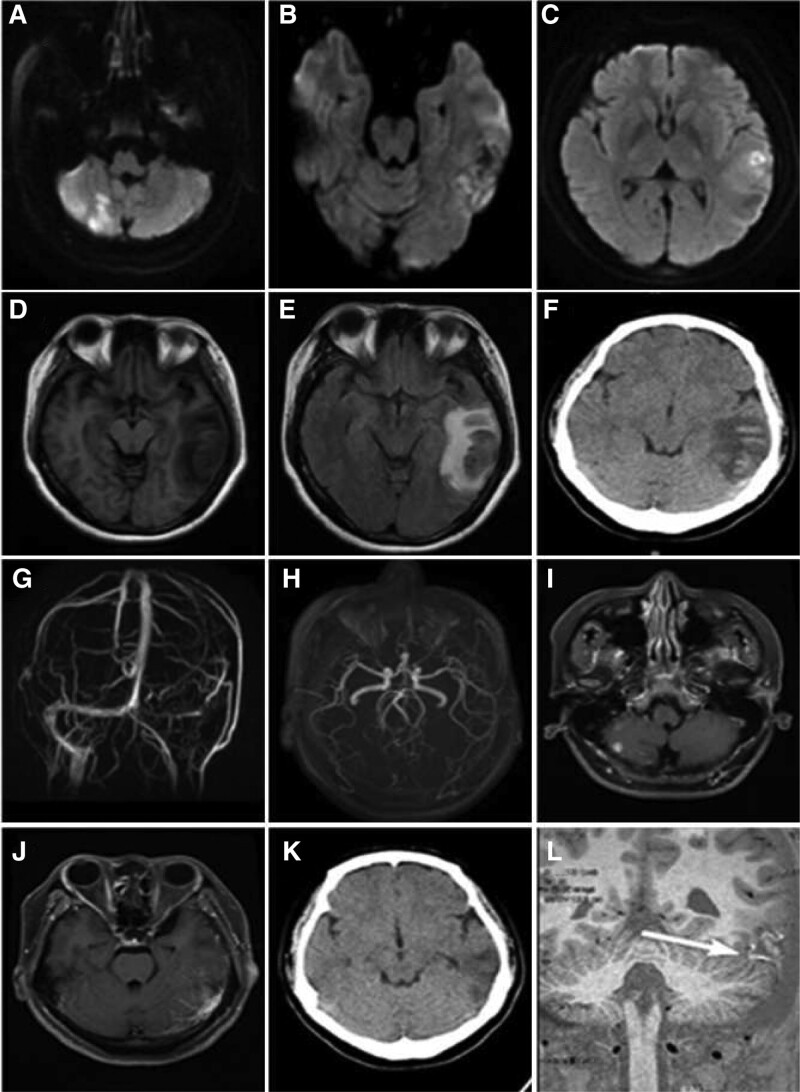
Brain imaging findings before and after treatment. (A, B and C) Diffusion-weighted imaging showed multiple infarcts. (D and E) T1-weighted images (T1WI) and Fluid-attenuated inversion-recovery (FLAIR) images showed heterogeneous signal and distinct edema in the left temporal lobe. (F) CT scan showed hemorrhagic infarction in the left temporal lobe. (G) Magnetic resonance venography (MAV) demonstrated a vague left sigmoid sinus. (H) Magnetic resonance angiography (MRA) showed no arterial vascular stenosis or occlusion. (I and J) Cranial contrast-enhanced MRI revealed cord-like enhanced signals in the left temporal lobe and a strong signal in the left sigmoid sinus. (K) Follow-up CT scan showed the disappearance of high density in the left temporal lobe after a month. (L) High resolution MRI with T1WI 3D-VISTA displayed chronic thrombosis in the left sigmoid sinus, white arrow represented sigmoid sinus. MRI = magnetic resonance imaging.
